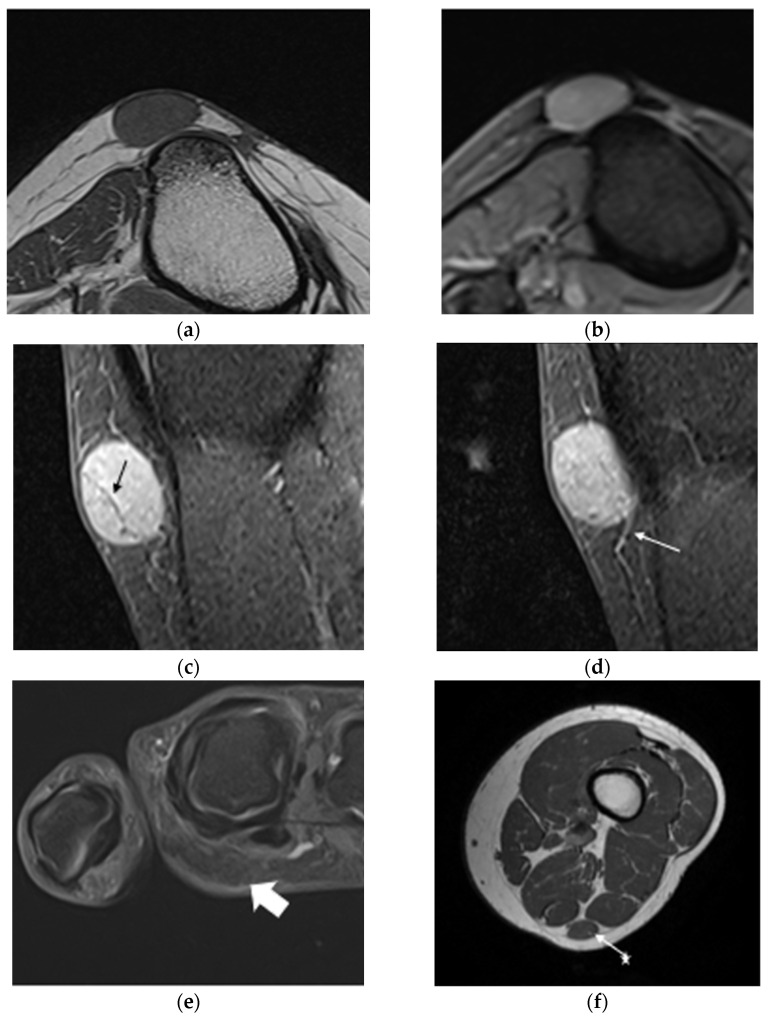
Magnetic resonance imaging characteristic of angioleiomyoma: common appearance of angioleiomyomas in a 50-year-old woman (a–d). (a) Axial T1-weighted MRI image of the left leg, demonstrating the subcutaneous low signal intensity of the lesion with well-defined low signal intensity margins located near the anterior tibial tuberosity but not attached to the periosteum. (b) Axial T2 gradient-echo sequences image showing a high signal intensity. (c) Sagittal fat-suppressed gadolinium T1-weighted image showing high contrast uptake with a reticular sign (black arrow). (d) The same sequence and cut plane as (c) but further cut showing high contrast uptake with afferent vascularization (white arrow). (e) Atypical presentation of axial T2-weighted fat-saturated image showing an iso-signal intensity (n = 3/78) located in the palmar region of the index finger of the right hand (white arrow). (f) Axial T1-weighted, subaponeurotic iso-signal lesion located between the semitendinosus and biceps femoris muscles of the left thigh (white arrow).
